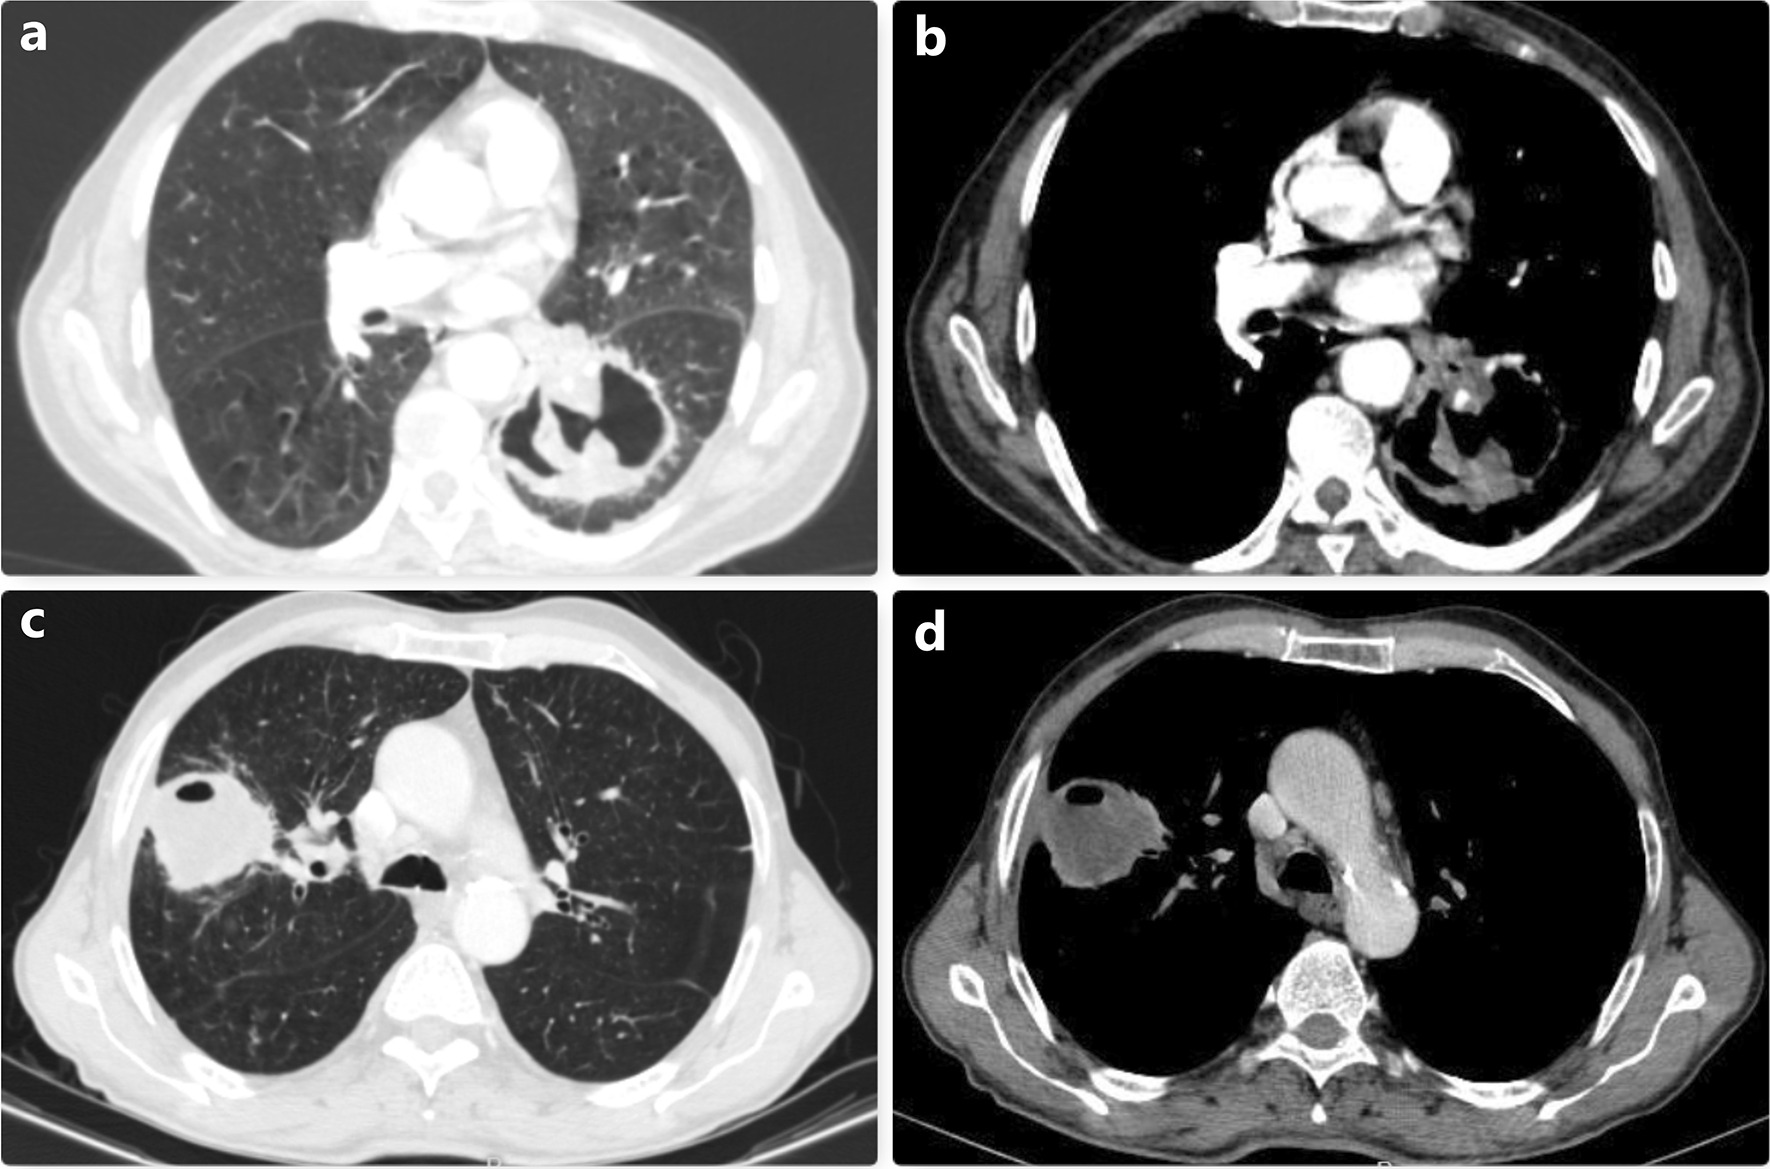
In (a) and (b), thorax CT images of the cavitary lesion located in the left lower lobe are observed. In this patient who was operated with a preliminary diagnosis of hydatid cyst, the diagnosis was made as squamous cell carcinoma and lobectomy was performed. (c) and (d) show a cavitary lesion located in the right upper lobe. In this patient, squamous cell lung cancer was diagnosed intraoperatively, and upper lobectomy was performed
